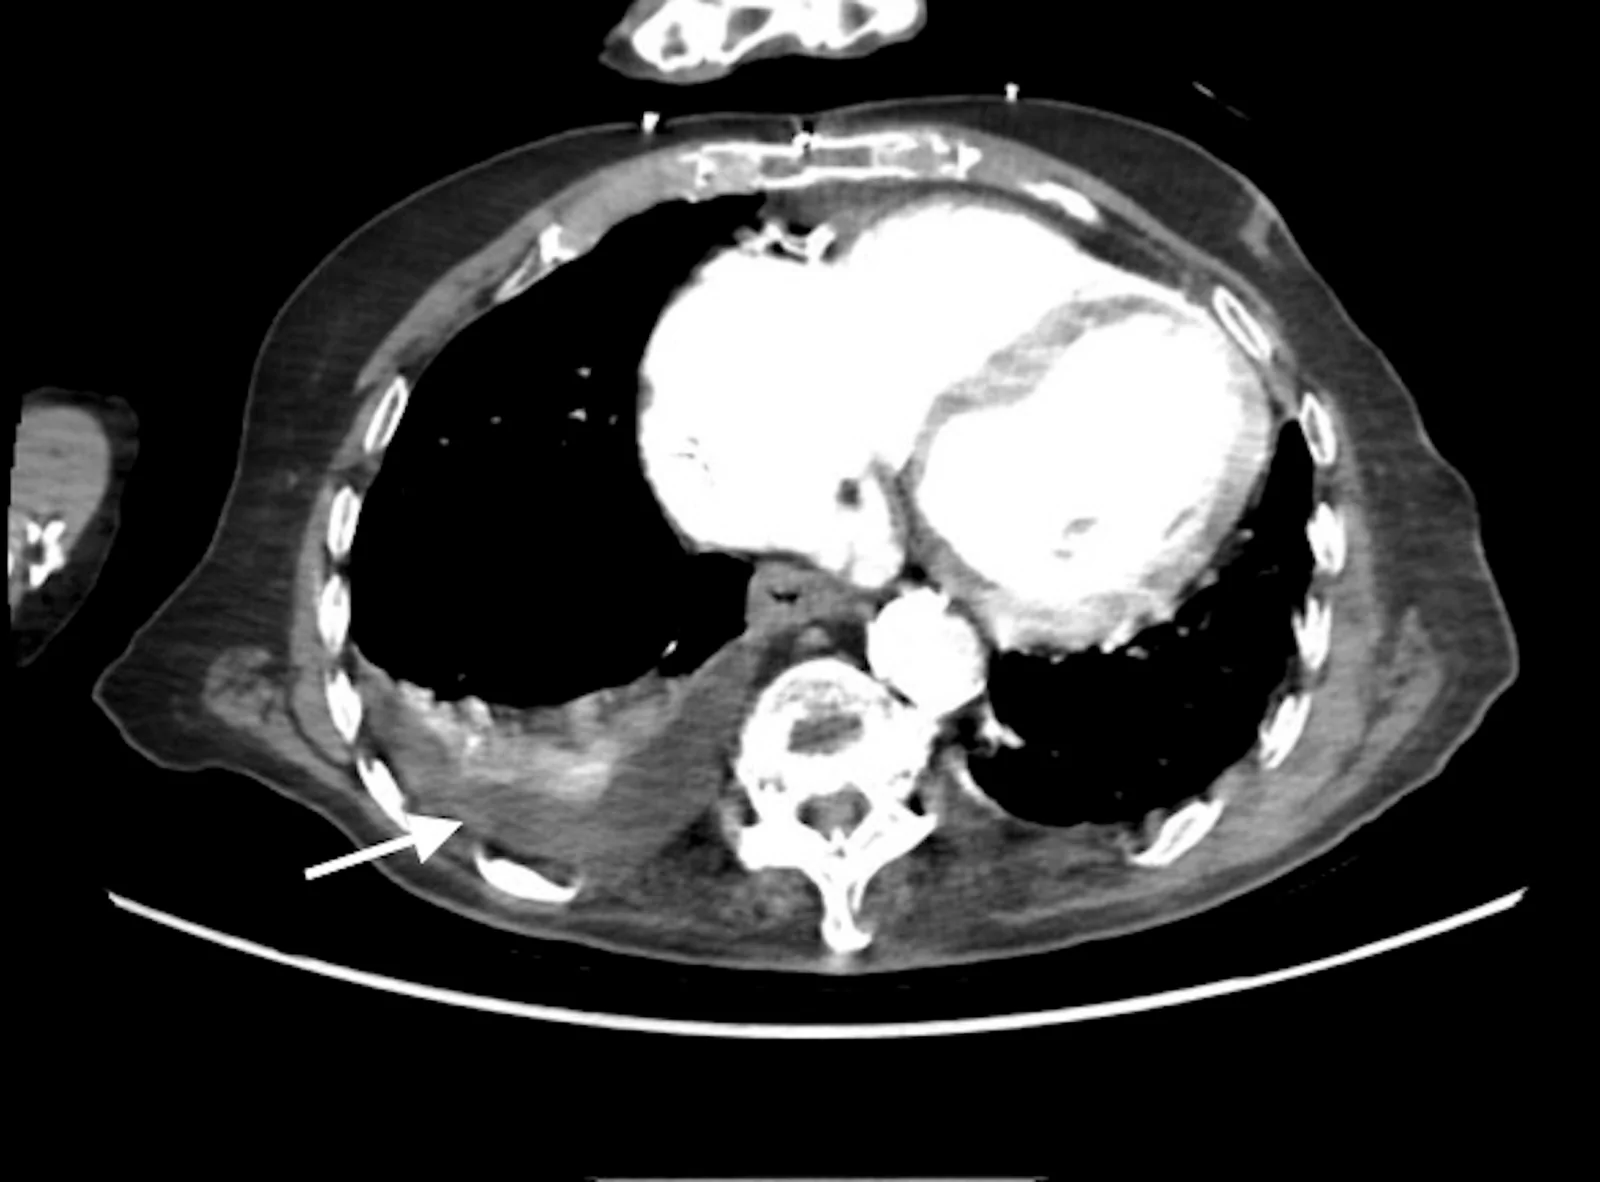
CT image of the chest demonstrating a right pleural effusion with a possible hemothorax.
